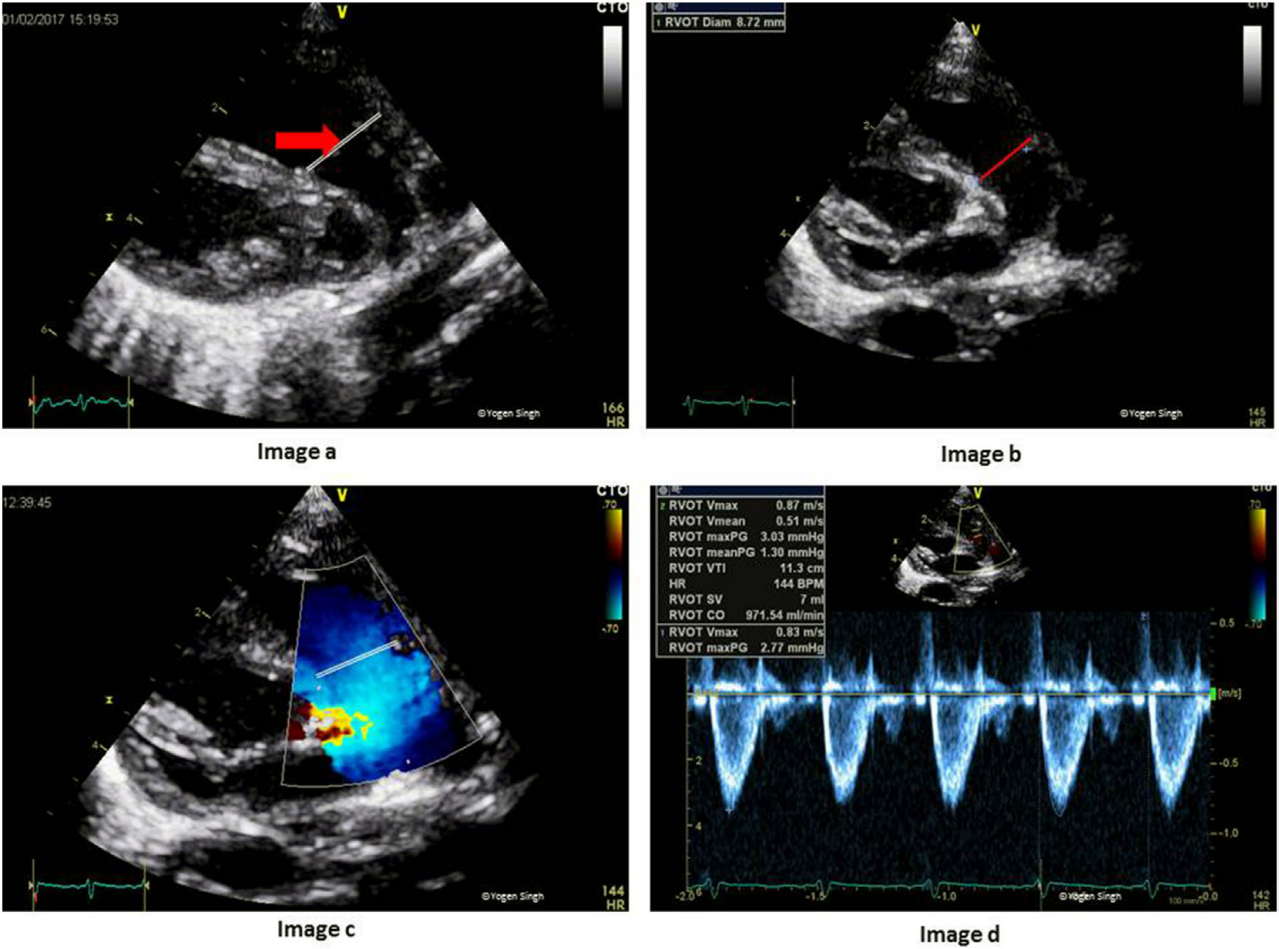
Assessment of right ventricular output (RVO) on echocardiography. Image a shows RV outflow tract (red arrow) and image b with measurement of PV annulus (red line) at the hinge point of PV valve. Image c shows RV outflow tract and the site for PW Doppler to measure VTI (red line showing PW sample gate). Image d shows RVO in milliliters per minute. PV, pulmonary valve; PW, pulse wave; RV, right ventricle; VTI, velocity time integral.
